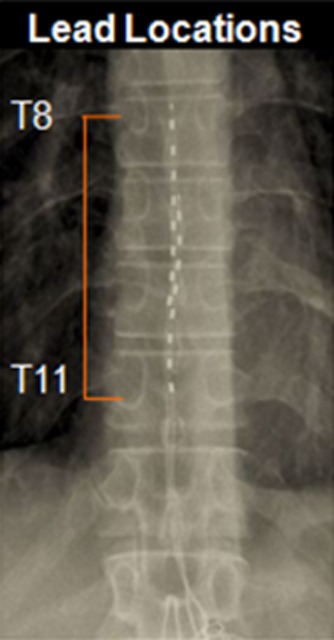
X-ray of leads spanning T8 to T11.
